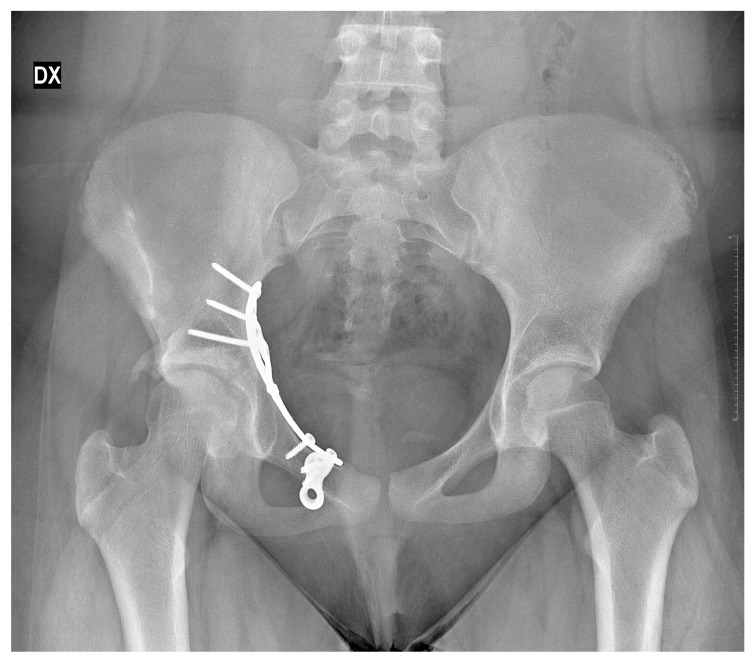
X-rays follow-up at 30 months by the surgical treatment. DX = Right.
